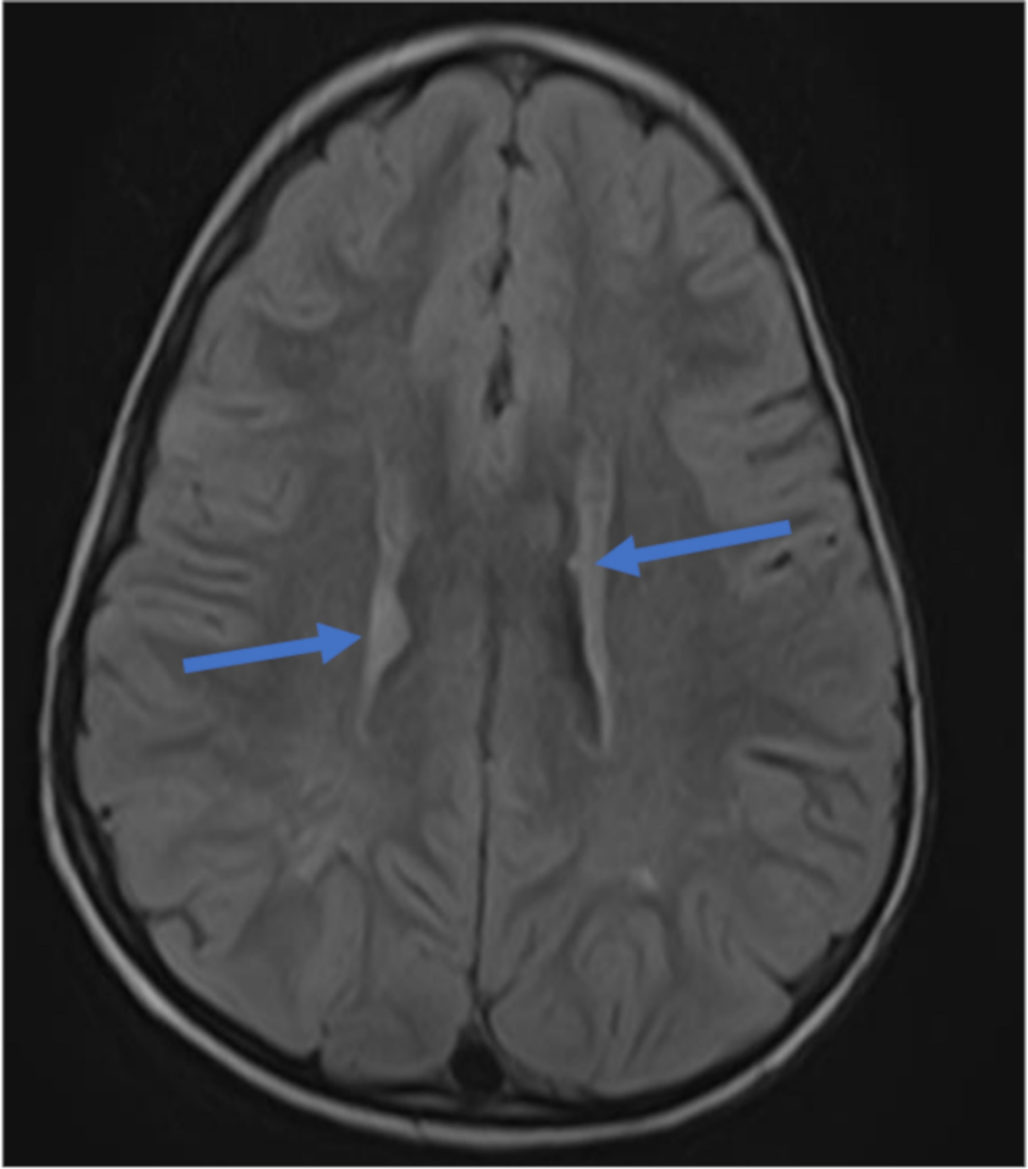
MRI, Axial FLAIR showing focal thickenings/nodules along the subependymal region of the body of the lateral ventricles, measuring 1.5mm in diameter representing tiny subependymal nodules (blue arrows).
